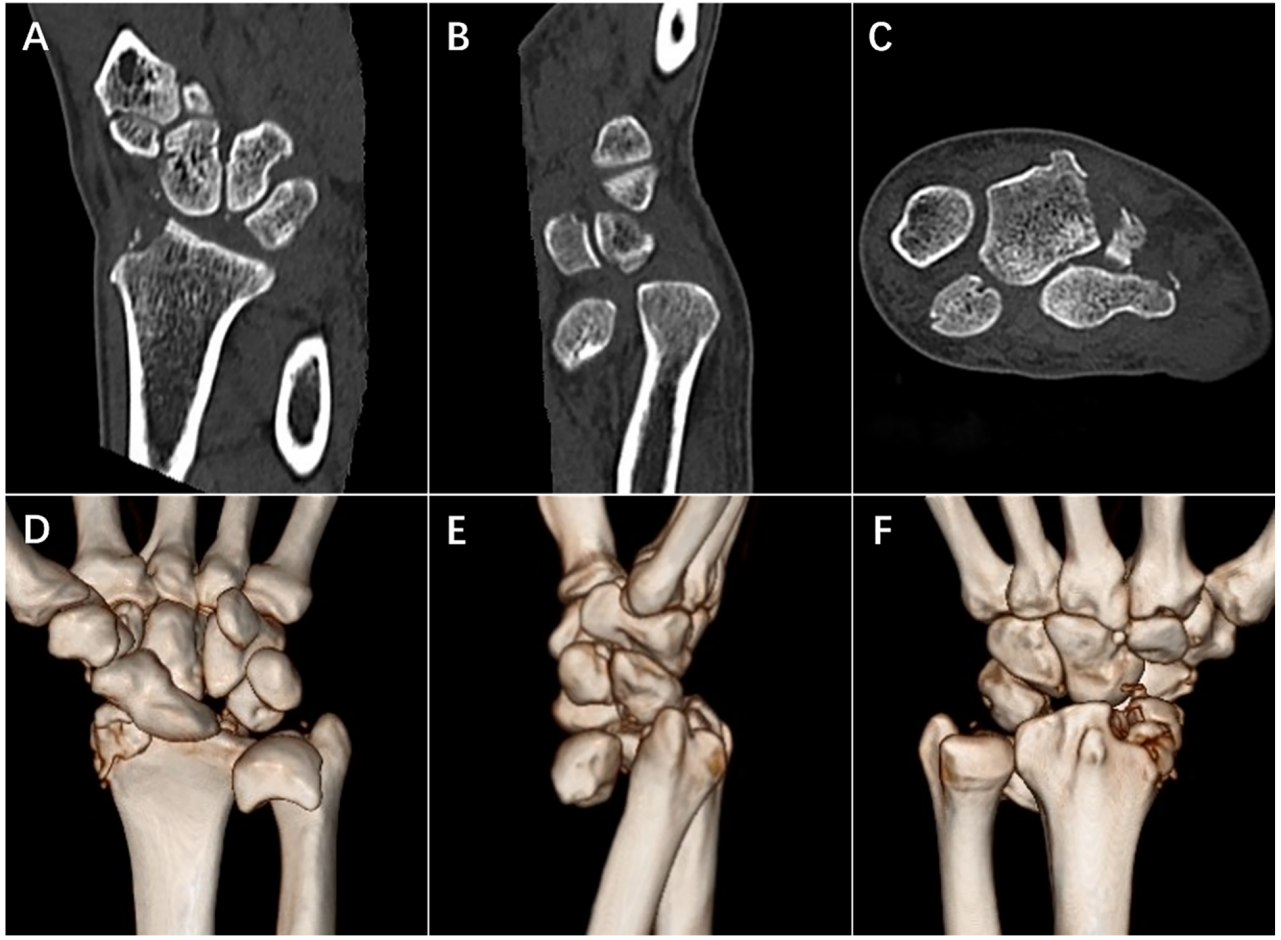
Pre-operative CT of injured left forearm. Coronal plane (A), sagittal plane (B) and transverse plane (C) of CT imaging showed dislocation and separation of lunate and scaphoid as well as multiple fracture. 3D reconstruction results (D–F) showed injury in details.
